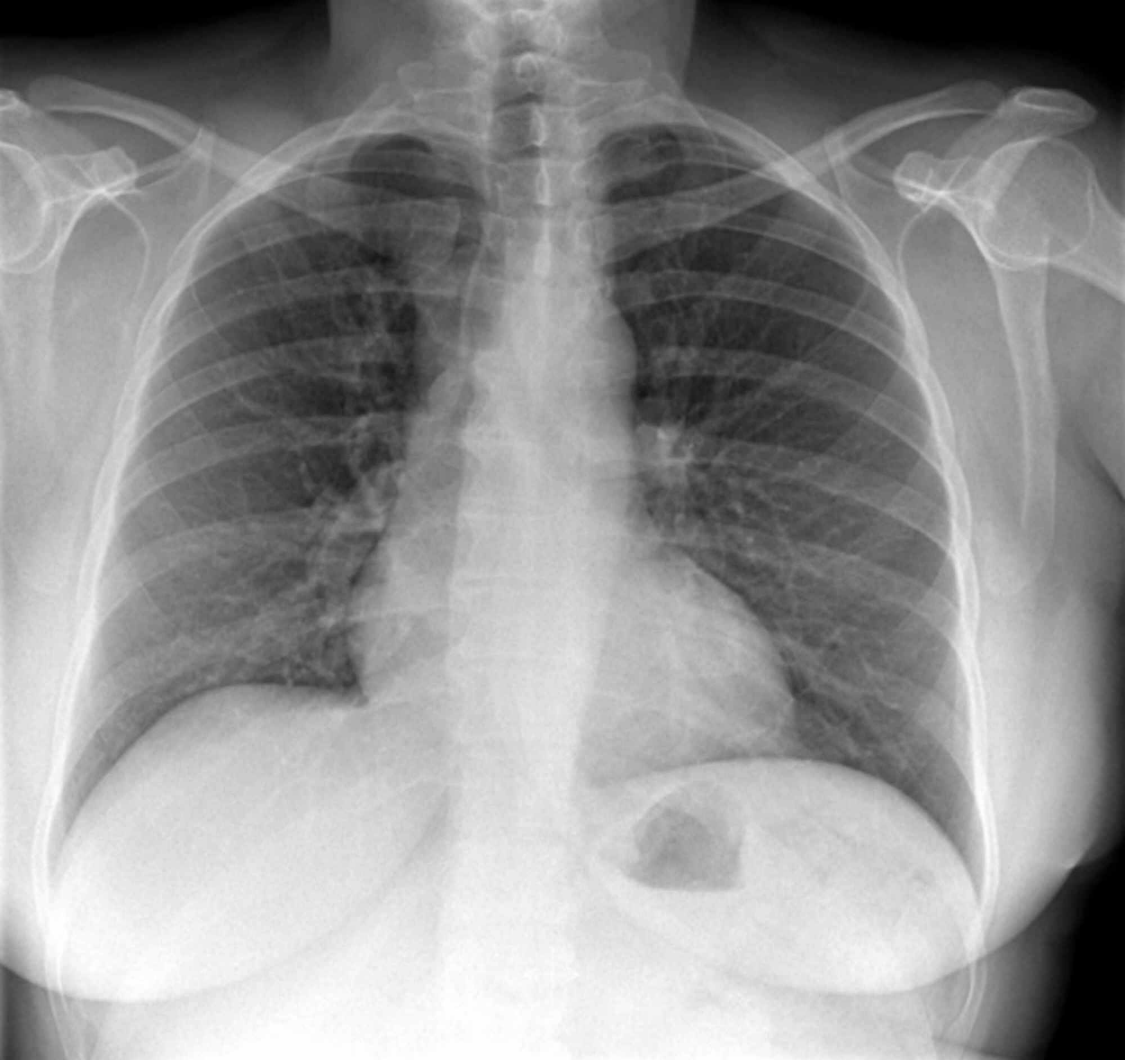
A follow-up chest X-ray three months after surgery shows complete disappearance of the pleural effusion.
